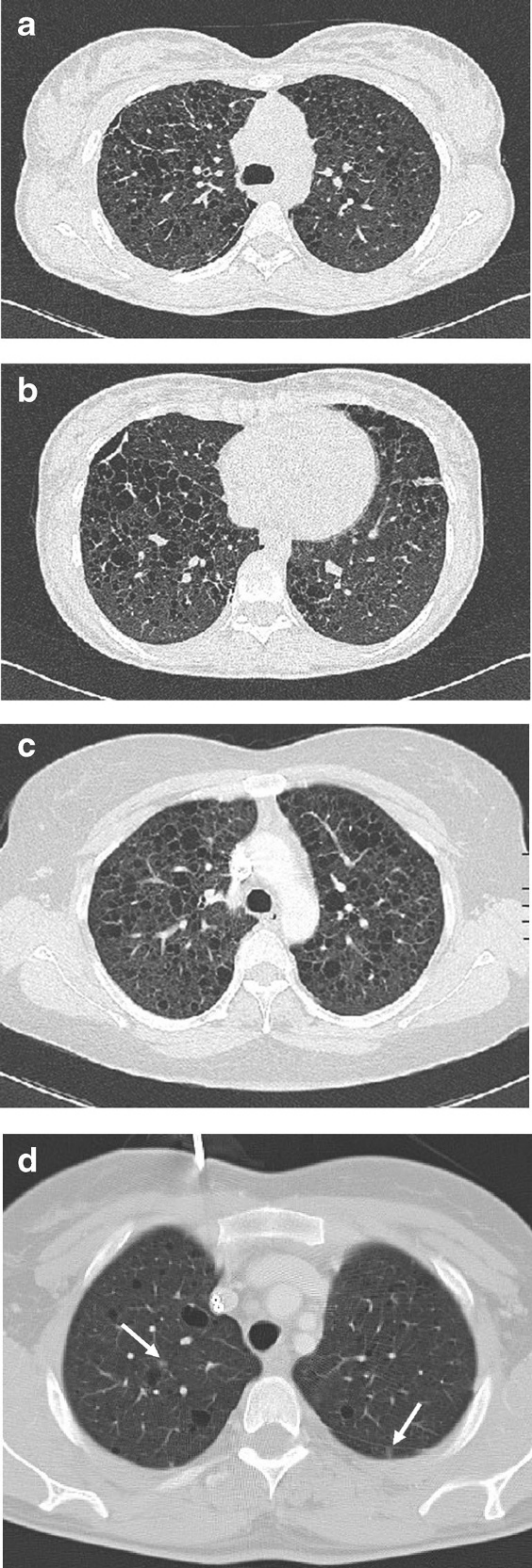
Cystic PLCH compared with LAM a, b A 22-year-old woman hospitalised for pneumothorax. Medical history was negative for respiratory disease. Definitive diagnosis of PLCH obtained by VATS performed within a month of imaging. HRCT scans show numerous thin-walled cysts of mostly ovoid and polygonal shape with a nearly imperceptible predominant craniocaudal distribution. Persistence of a small pneumothorax is shown. c, d Biopsy-confirmed LAM in two young female patients. A 3-mm collimated CT scan shows diffuse (c) and mild (d) pulmonary involvement of thin-walled cysts randomly distributed throughout both lungs, with some faint micronodules (*arrows*) (compare d with Fig. 1)
